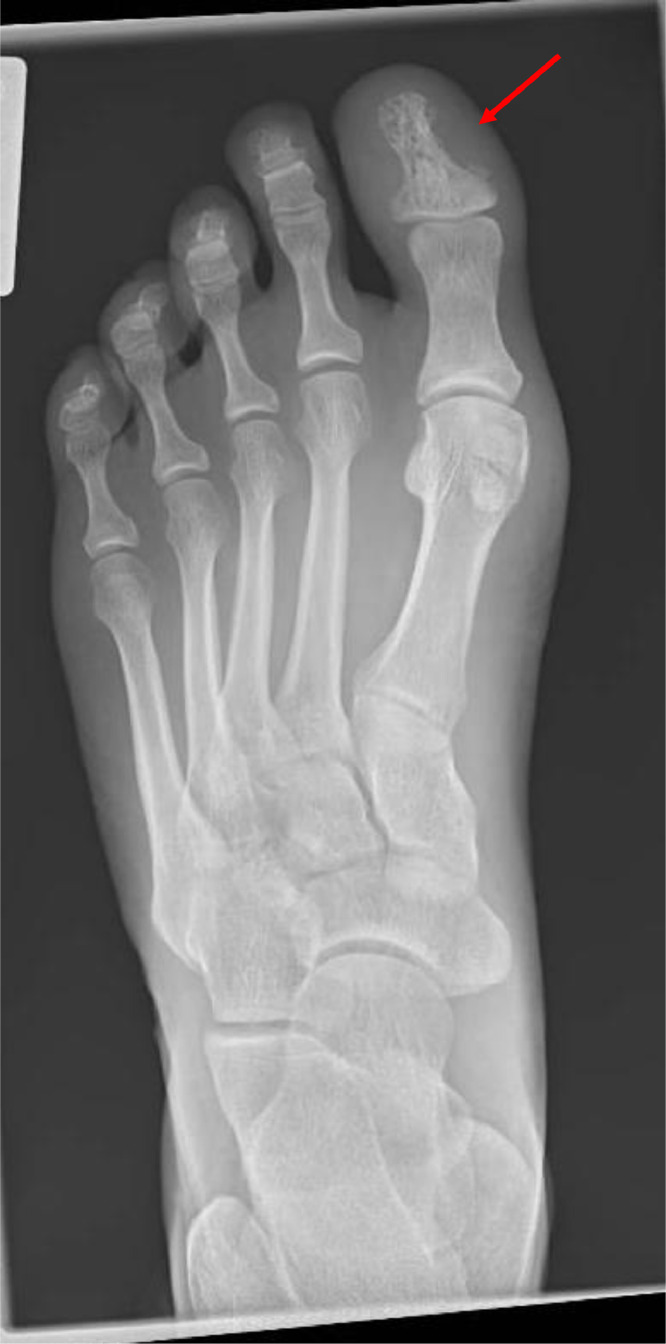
Radiographic appearance of sarcoidosis affecting bone. Typical radiographic appearance of bone involvement by sarcoidosis: lace-like honeycomb appearance of sarcoidosis involving first metatarsal (arrowed) on plain radiograph.
